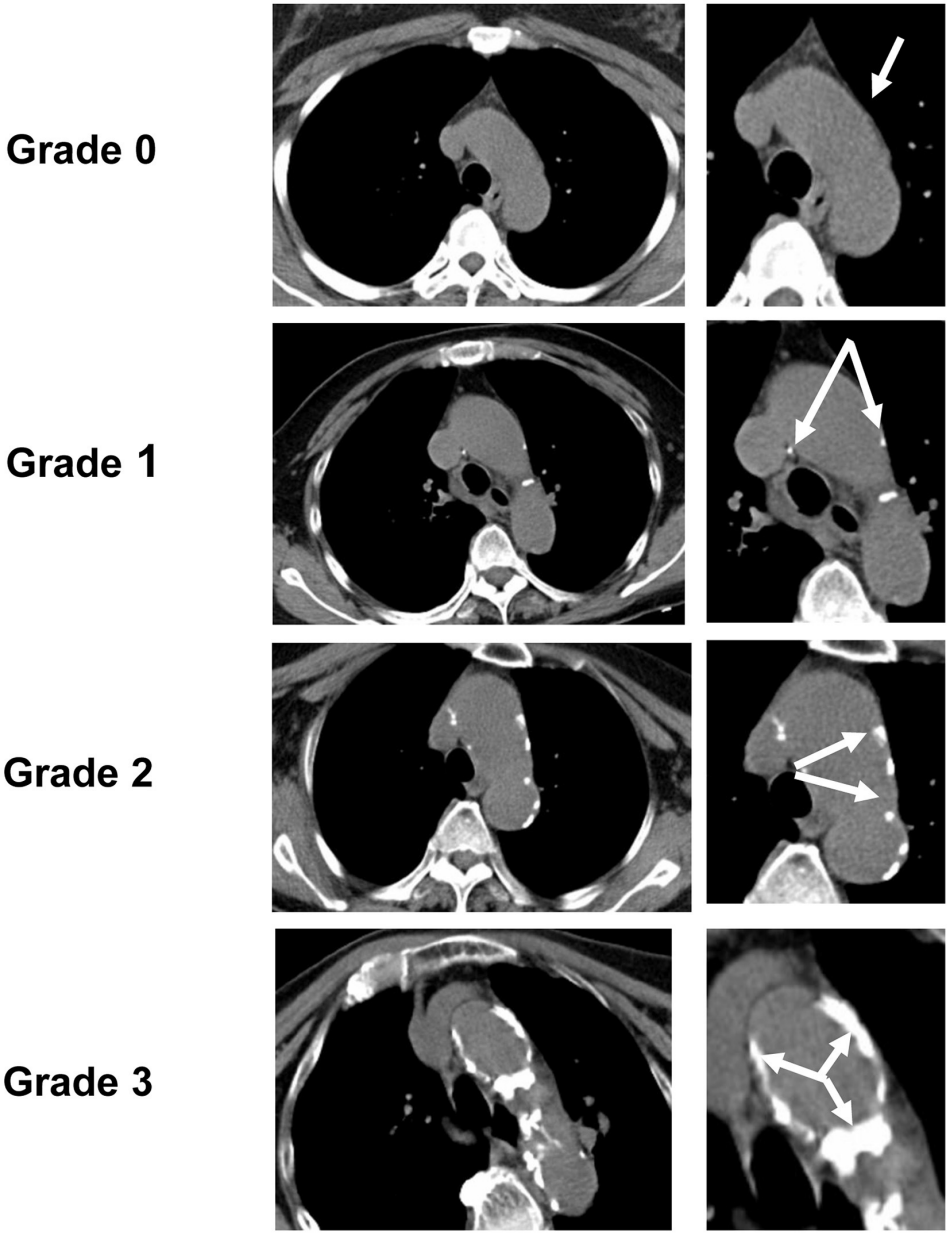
Aortic arch calcification grading scale (AGS). According to AGS, the degree of aortic arch calcification (AoAC) detected by chest CT was divided into four grades (the white arrow points to the calcification area): grade 0, no visible calcification; grade 1, spotty calcification of the aortic arch ≤ 1 mm in diameter; grade 2, lamellar calcification > 1 mm in diameter; grade 3, circular calcification.
